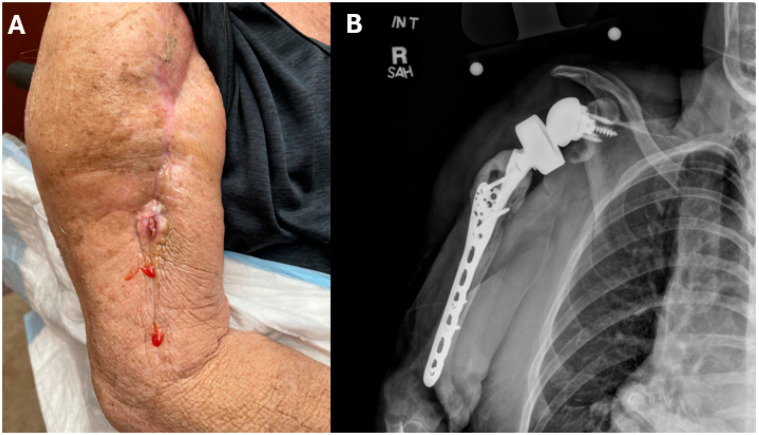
Right shoulder periprosthetic joint infection. (A) Image of right shoulder with purulent drainage at site of previous arthroplasty scar. (B) X-ray of right shoulder demonstrating significant soft tissue swelling.
